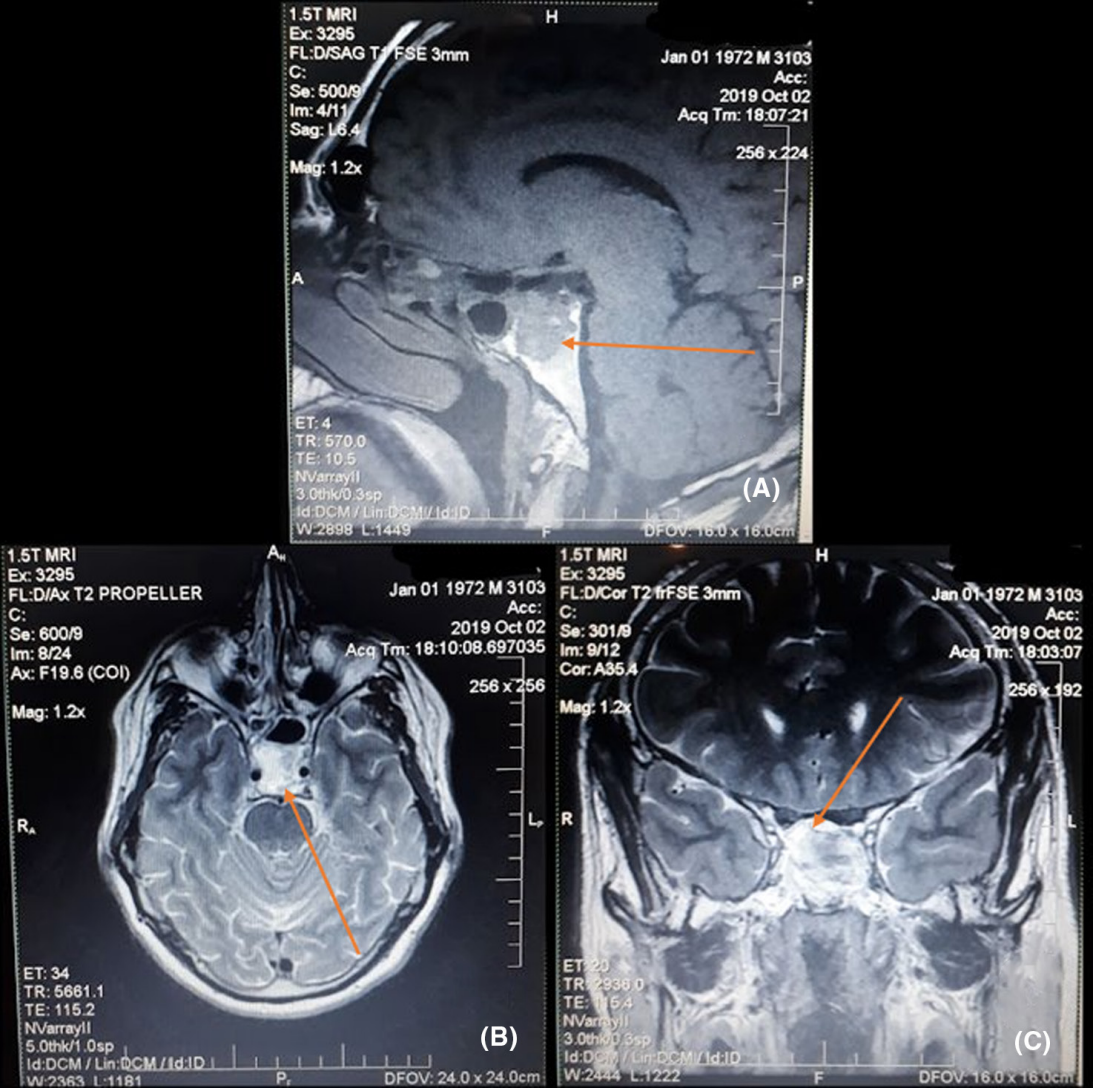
(A, B, and C): MRI with contrast of sphenoid sinus and pituitary gland in sagittal (A), transverse (B), and coronary (C) sections showing a mass in the clivus with moderate enhancement and peripheral calcifications.
